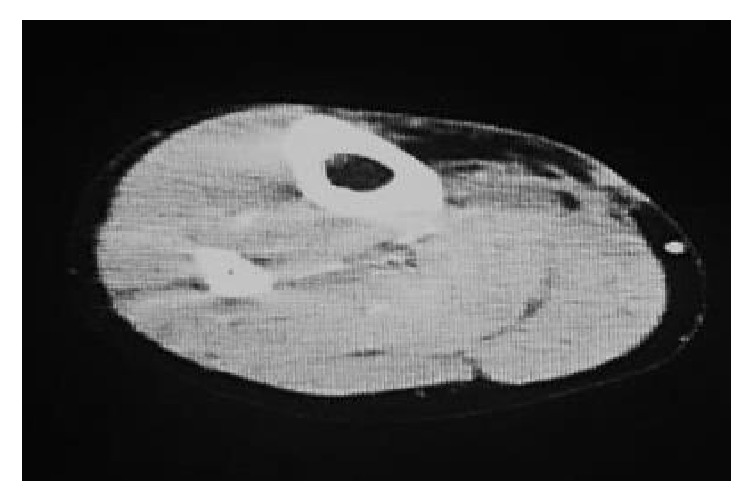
CT on day 100. Swelling was alleviated and disappearance of abscess was confirmed.
